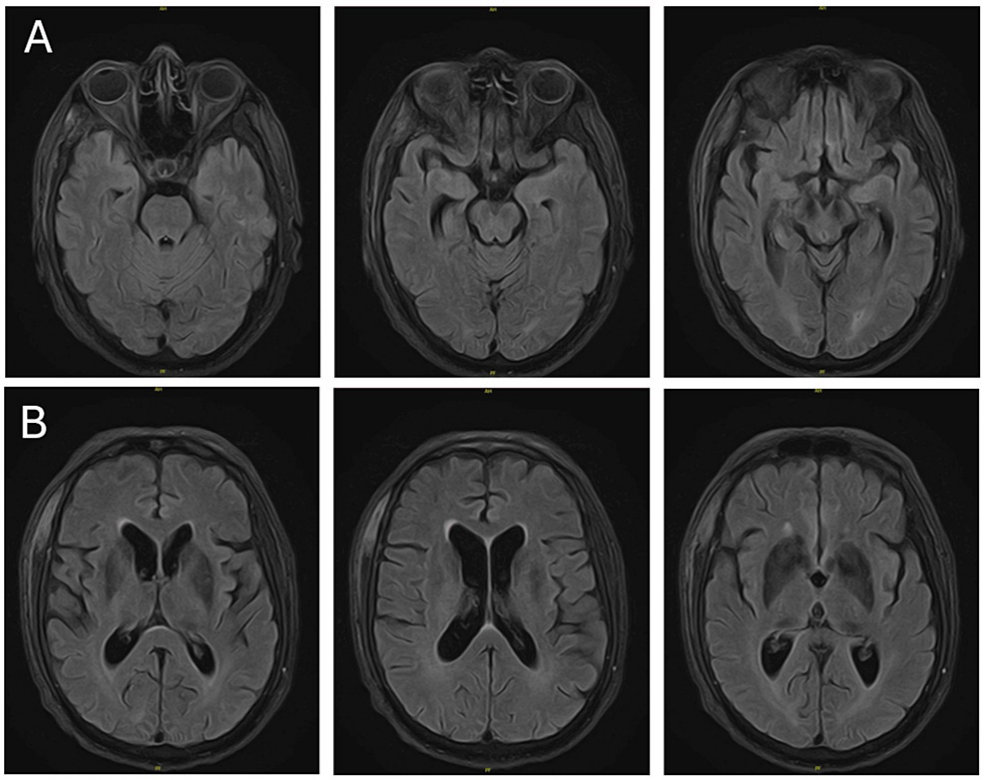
Axial T2-FLAIR view of the brain MRI with non-specific white matter disease but specifically demonstrating absent signal intensity changes in the hippocampus and the basal ganglia A) Serial axial T2-FLAIR images displaying the hippocampus; B) Serial axial T2-FLAIR images displaying the basal ganglia MRI: magnetic resonance imaging; FLAIR: fluid-attenuated inversion recovery
